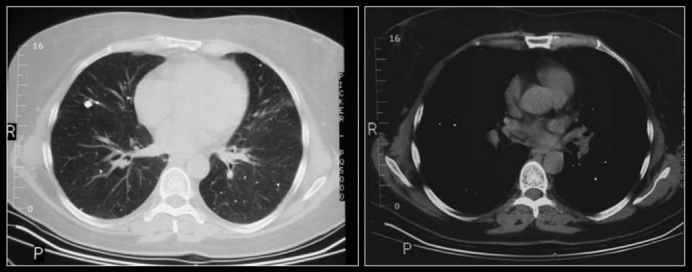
Axial CT scans shows multiple small calcific PNs due to old healed histoplasmosis
